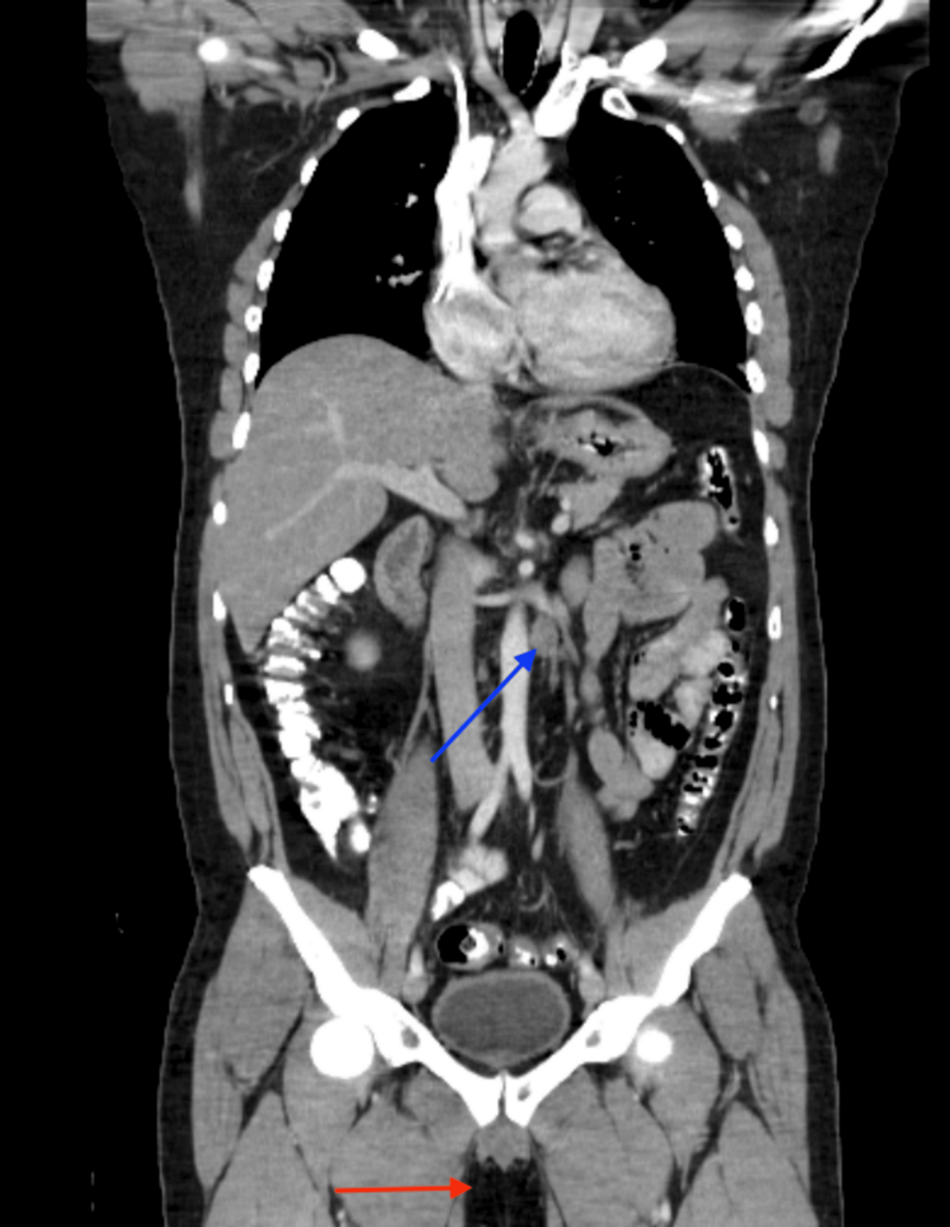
Computed tomography (CT) scan of the chest, abdomen, and pelvis showing improvement (red arrow with no testicle and blue arrow showing a decrease in the para-aortic lymph nodes).
